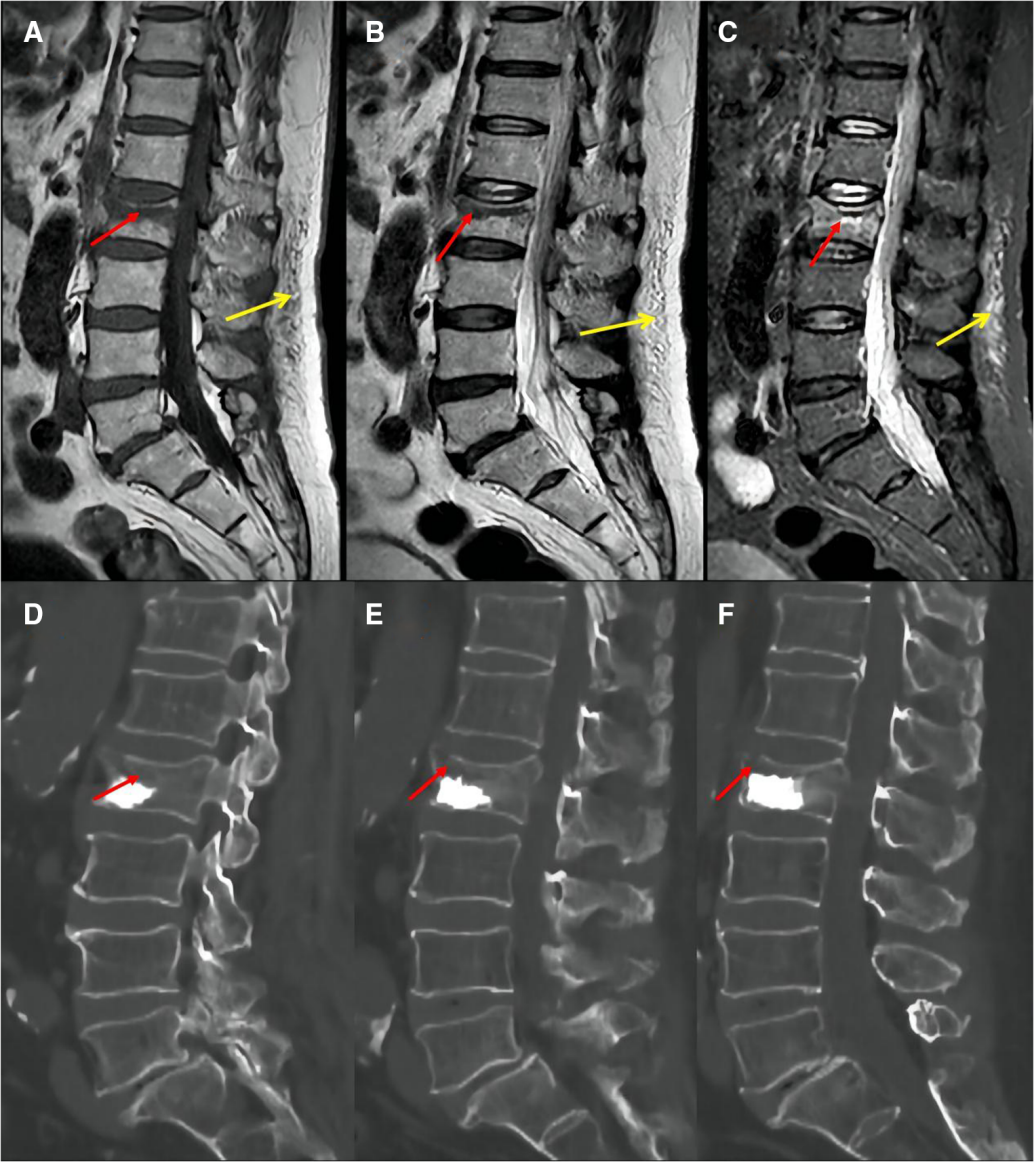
Preoperative MRI images showed that the fracture area was located in the upper third of the vertebral body (A: T1, B: T2, C: STIR, red arrow); postoperative CT images showed insufficient cement filling in the vertebral fractured area (D: left in median sagittal section; E: median sagittal section; F: right in median sagittal section, red arrow). Posterior fascia edema:hypointense on T1-weighted images (A, yellow arrow); hyperintensity on T2-weighted images (B, yellow arrow) or STIR images (C, yellow arrow).
